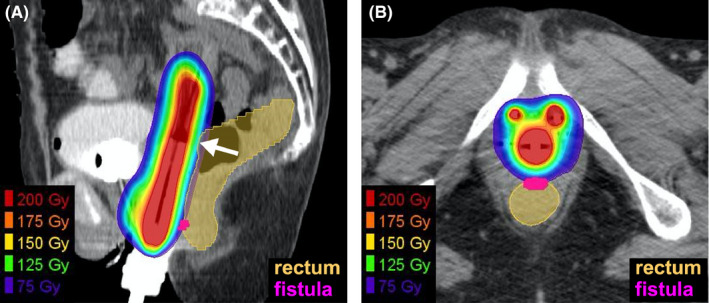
Distribution of the total equivalent dose in 2 Gy‐fractions, with the α/β ratio of 3 (EQD2_3_) from external beam radiotherapy (EBRT) and brachytherapy superimposed on the treatment planning computed tomography images for brachytherapy (third session). (A) Sagittal plane. (B) Axial plane. The arrow indicates the part of the rectum in which the total EQD2_3_ exceeded 75 Gy. EBRT plan was created using Eclipse (Varian, Palo Alto, CA, USA). Rigid registration of EBRT‐ and brachytherapy‐plans and calculation of the total EQD2_3_ were done using MIM Maestro version 6.9.3 (MIM Software Inc, Beachwood, OH, USA)
